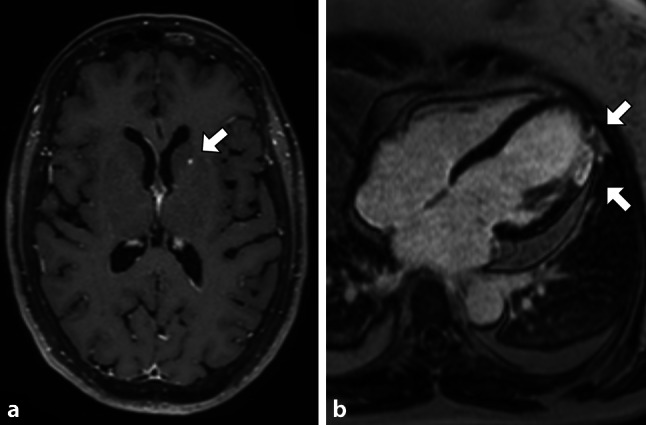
Magnetic resonance imaging. a Section of cerebrum showing embolism in left internal capsule (*see arrow*). b Section of heart with transmural apical late enhancement with microvascular obstruction (*see arrows*) corresponding with recent transmural infarction
